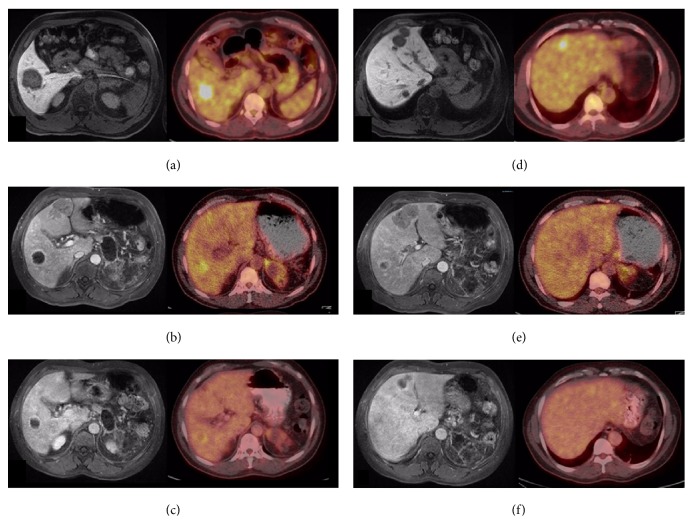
Magnetic resonance (MR) and positron emission tomography-computed tomography with 18-fluorodeoxyglucose (18-FDG PET-CT) imaging. (a–c) Axial MR images in the portal phase (left column) and axial 18-FDG PET-CT images (right column) showing the evolution of the largest hypovascular and hypermetabolic liver nodule in the* right lobe*: (a) at admission, (b) 30 days after the first SIRT session, and (c) 45 days after the second SIRT session. (d–f) Axial MR images in the portal phase (left column) and axial 18-FDG PET-CT (right column) showing evolution of the largest hypovascular and hypermetabolic liver nodules in the* left lobe*: (d) at admission, (e) 30 days after the first SIRT session (note that the lesions had grown), and (f) 45 days after the second SIRT session.
